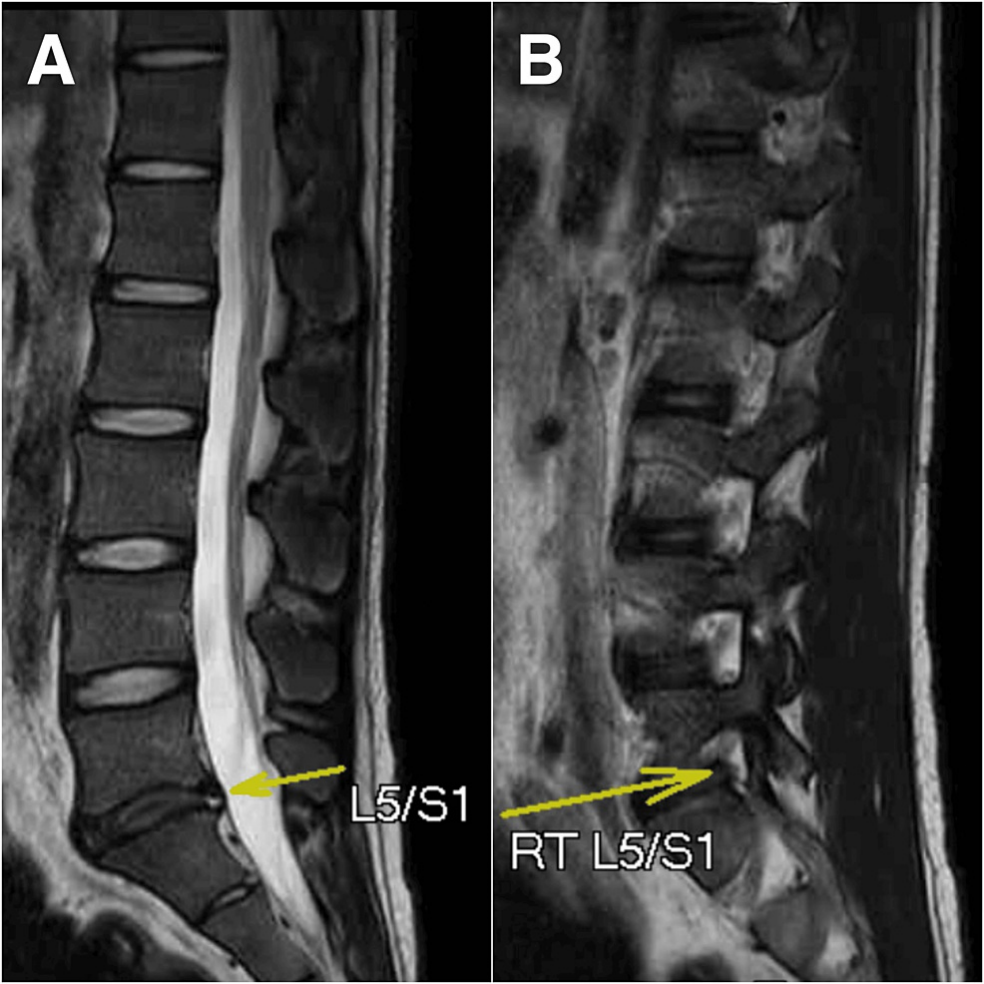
Sagittal view of magnetic resonance image (MRI) of lumbar spine A) MRI showing disc desiccation with decreased disc height noted at L5/S1 level (yellow arrow). A posterior annular tear can be observed at the L5/S1 disc. Disc desiccation with decreased disc height at L5/S1 level. A posterior annular tear is observed at the L5/S1 disc. B) Posterior disc bulging at L5/S1 level with impingement onto right S1 descending nerve root (yellow arrow).
